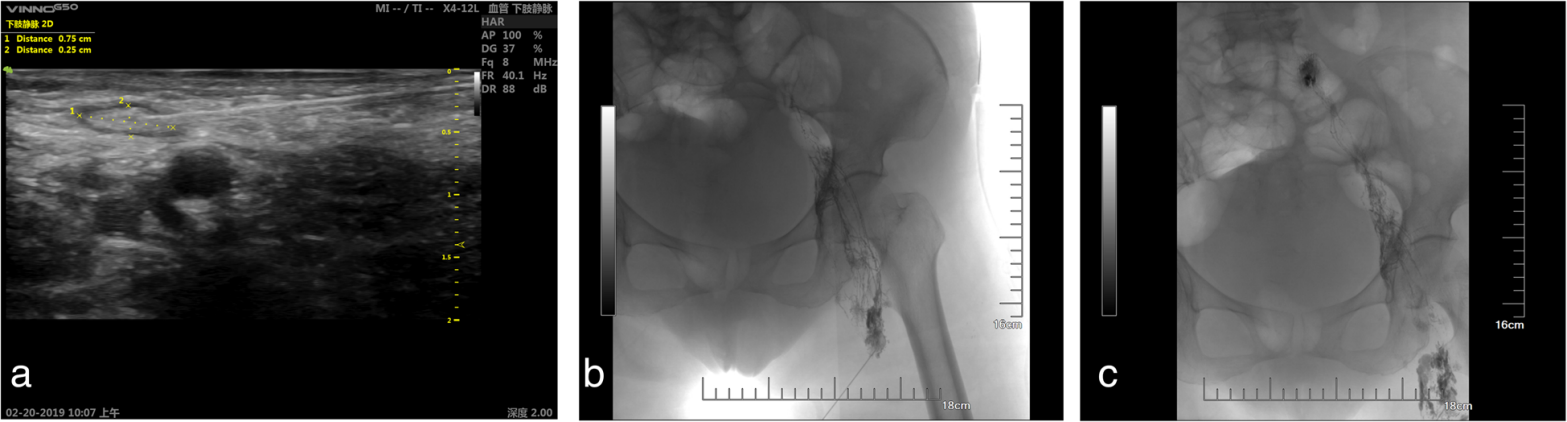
a Ultrasound-guided inguinal lymph node puncture. A 22-G puncture needle (white arrow) was guided by ultrasound to puncture the inguinal lymph nodes (black star). The circular hypoechoic area on the lower right is the femoral artery (white star). b Intranodal lymphangiography. The 22-G puncture needle (white arrow) punctured the inguinal lymph node (black star). The black arrows show the lymphatic vessel. c Lymphatic leakage embolisation. The white arrow shows the location of the lymphatic leakage. The black arrows indicate the path of the lymphatic vessel
